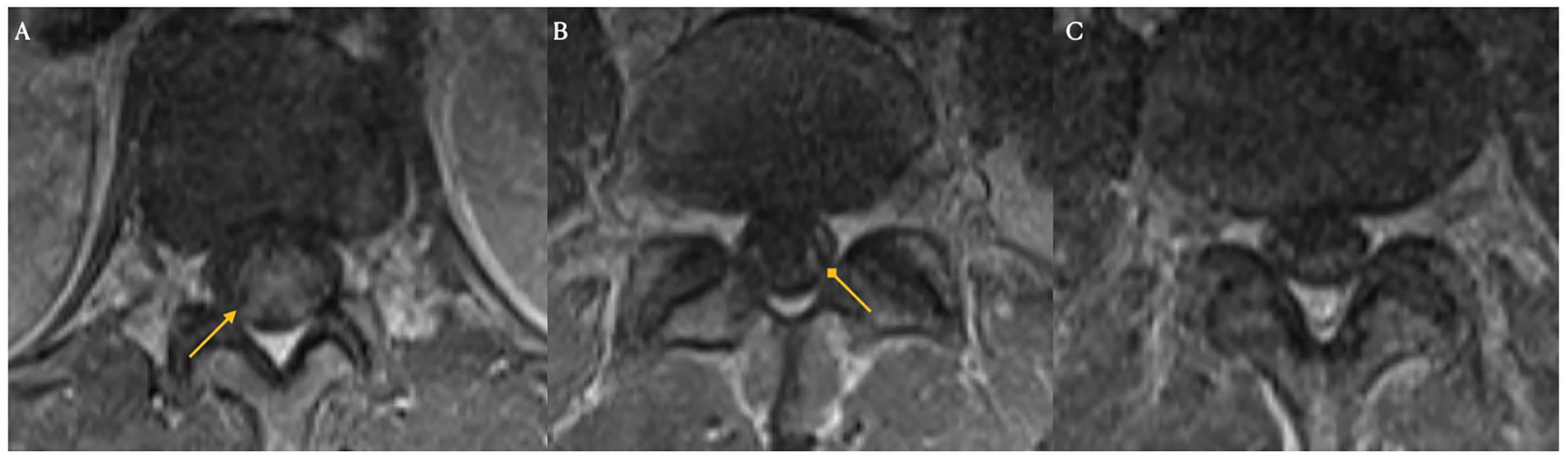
Spinal magnetic resonance imaging on day 21 post-treatment. New clumping and enhancement of the ventral cauda equina nerve roots observed on day 21 after treatment for ICANS and prophylactic IVIG (Panels A,B,C).
